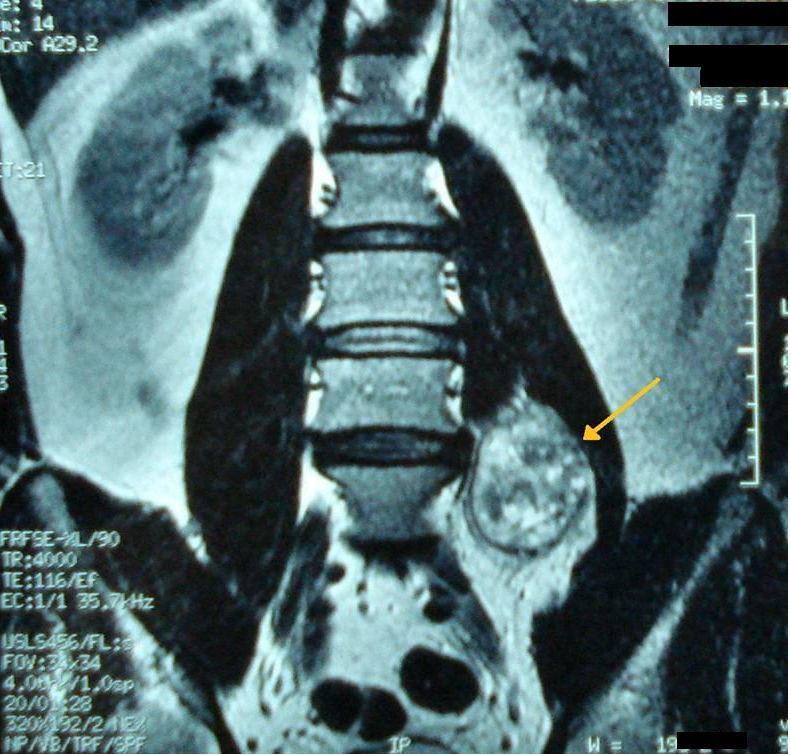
Abdominal MRI showing a well-defined encapsulated mass, in the left paraspinal region at the L5 level along the posterior side of left psoas muscle.
